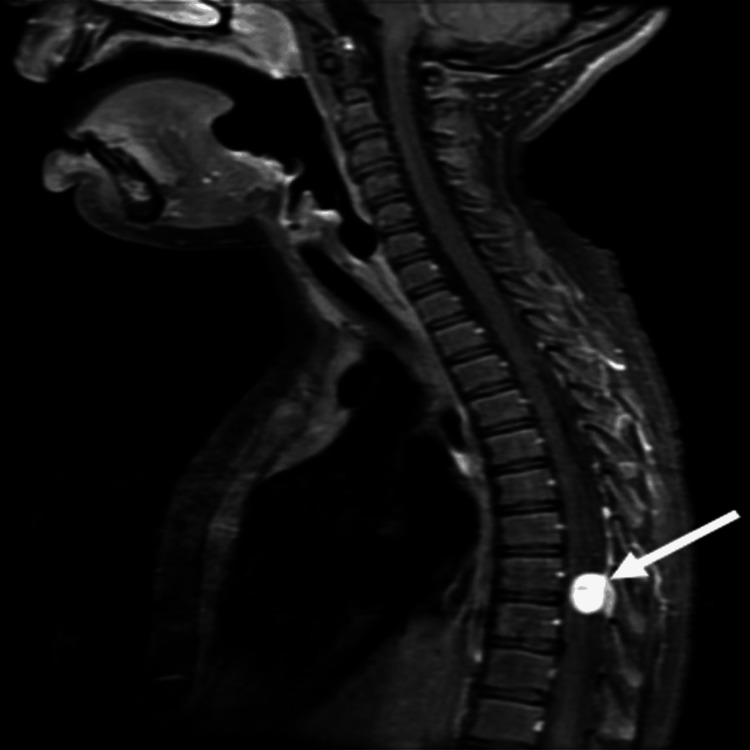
Spine MRI (T1 sagittal view) showing hypersignal intensity within the tumor site (arrow).
